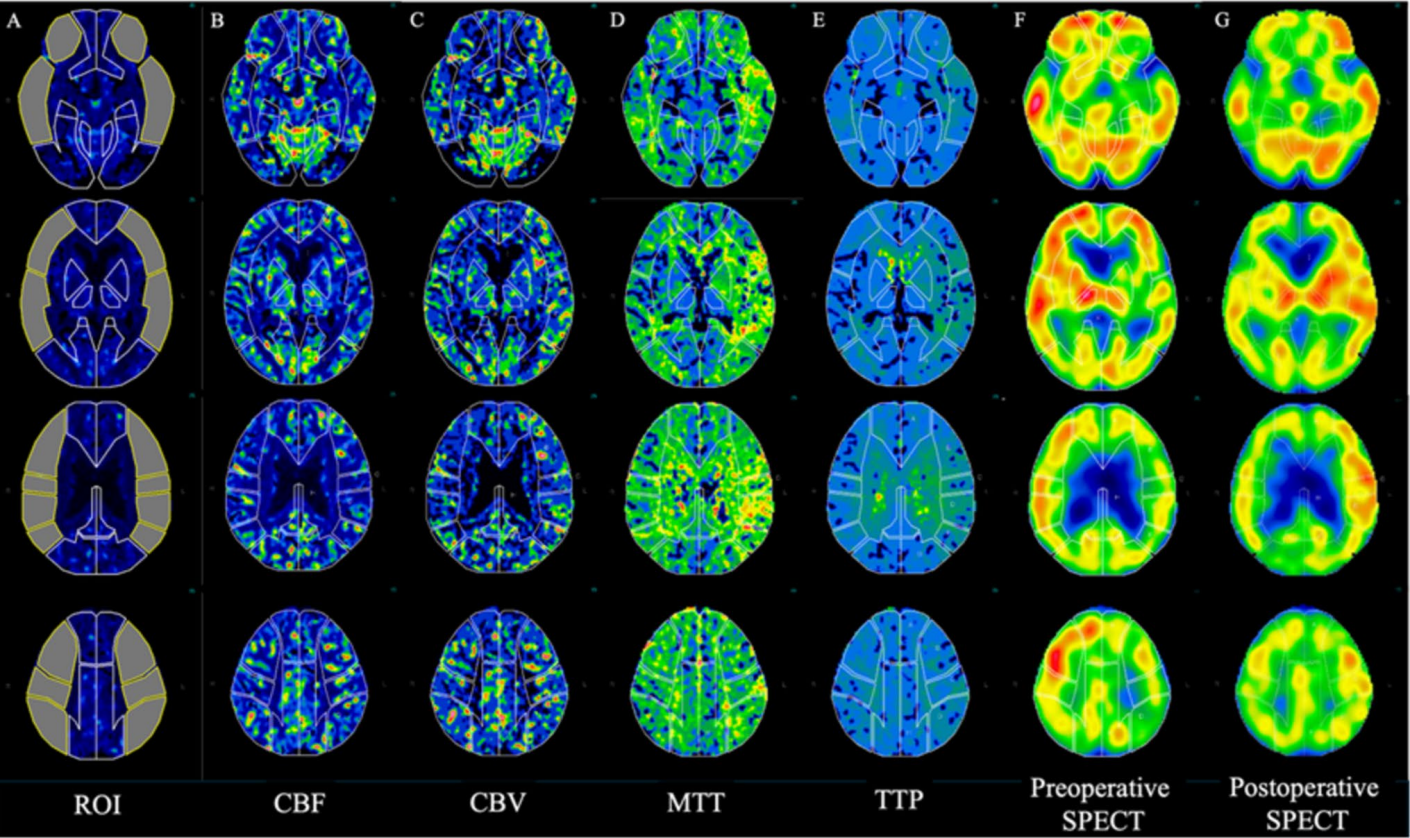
Reconstructed maps of CTP and ^123^I-IMP SPECT brain imaging at rest (case 10: left carotid artery stenosis). Regions of interest were automatically determined using 3D-SRT software, which converts volume data of CTP and ^123^I-IMP SPECT at rest to 59 slices of 2-mm interval standardized images. In this figure, 4 representative slices of 59 slices in each map of CTP parameter and ^123^I-IMP SPECT at rest are presented. A: yellow-circled ROIs were used for calculation as ROIs in the MCA territory, B: cerebral blood flow (CBF), C: cerebral blood volume (CBV), D: mean transit time (MTT), E: time to peak (TTP), F: preoperative ^123^I-IMP SPECT at rest, G: postoperative ^123^I-IMP SPECT at rest. The patient developed HPP without cerebral hyperperfusion syndrome including cerebral hemorrhage after CAS
